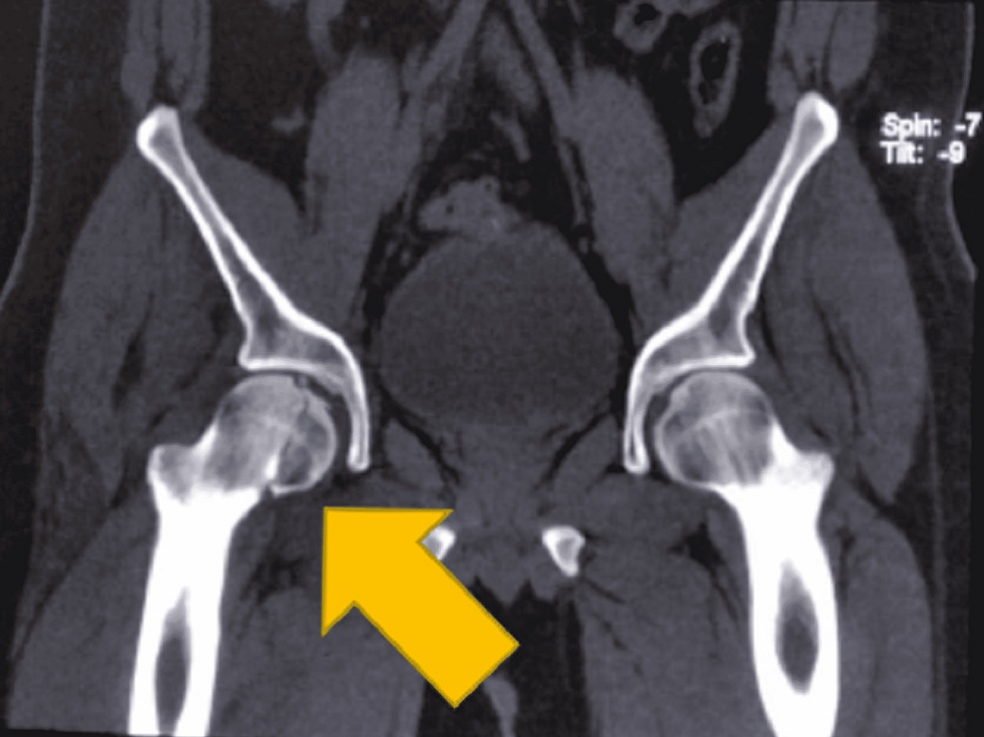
Coronal CT view showing Pipkin’s type II fracture.
